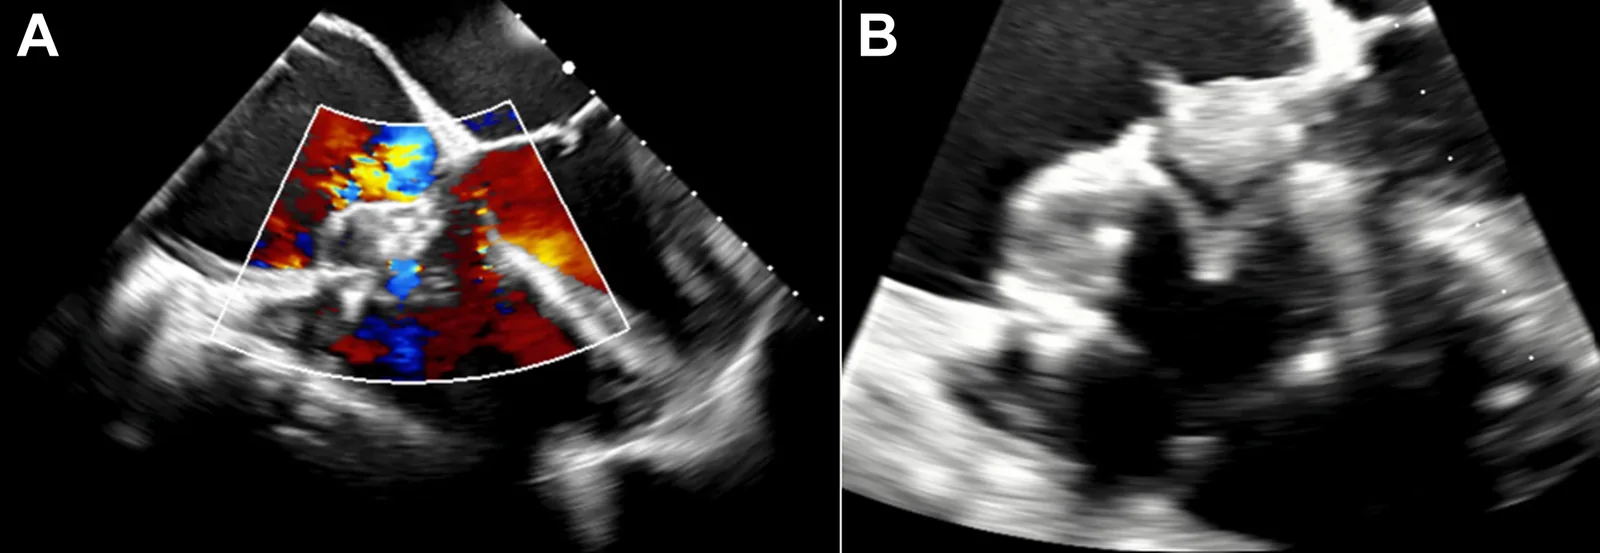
Transesophageal Echocardiography Shows Ventricular Septal Defect Created by the Embolized Evoque Valve (A and B) Ventricular septal defect created by the embolized Evoque valve.
